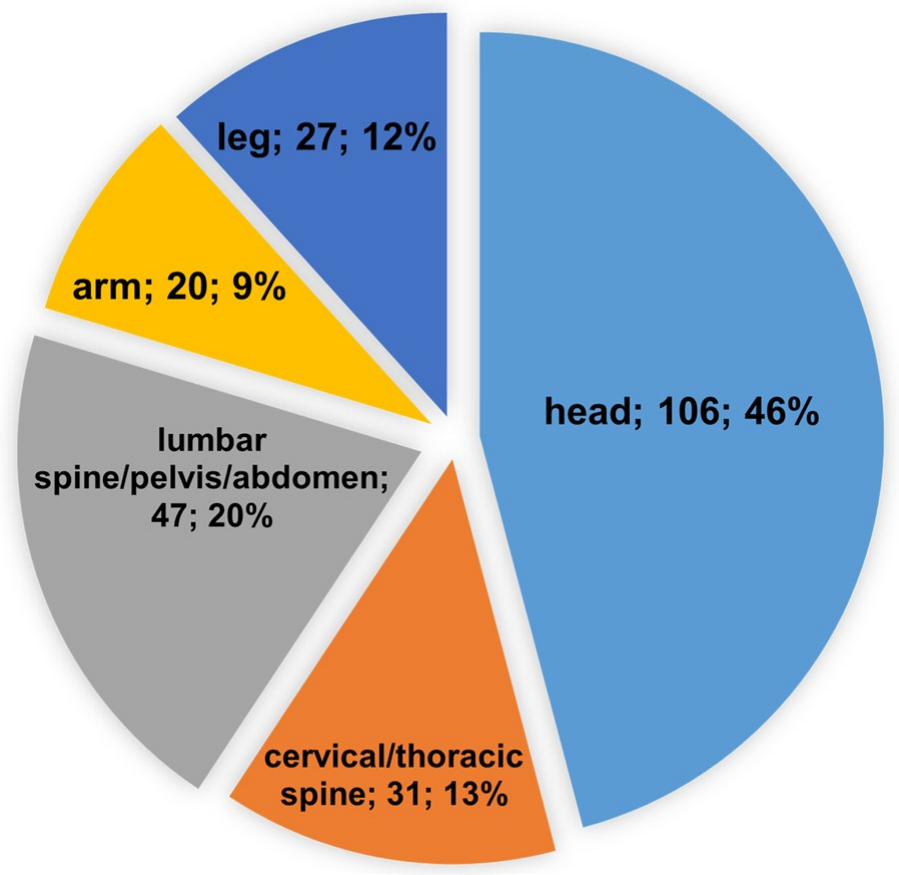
Body regions of all 231 CI exposures; body region scanned, number of MRI exposures, percentage of all MRI exposures; CI, cochlear implant; MRI, magnetic resonance imaging
